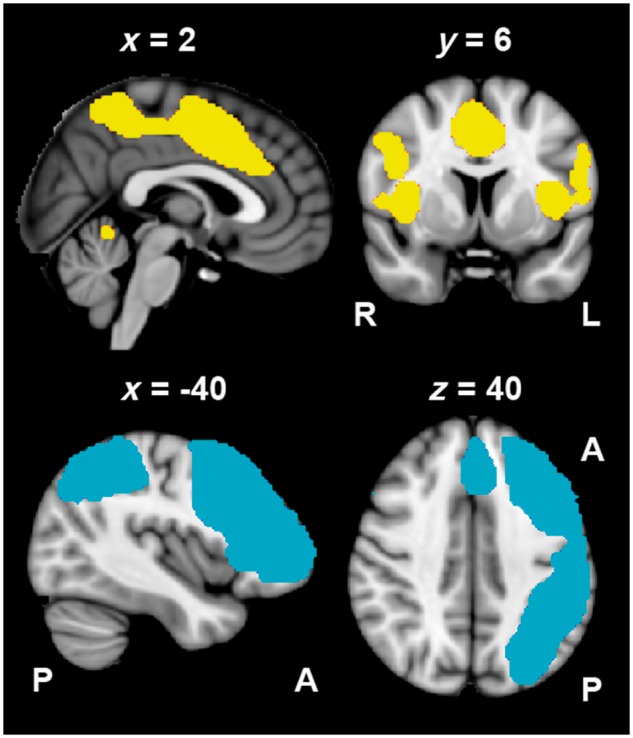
Standard T_1_-weighted anatomical slices overlaid with a mask of domain-general brain activity within cingular-opercular network (*top*) and a left-lateralized frontal-parietal-temporal language-specific network (*bottom*). The masks were obtained from previous analyses of control participants performing the same functional MRI task (Geranmayeh *et al.*, 2014b), and were used to calculate the lesion load in each network. R = right; L = left; A = anterior; P = posterior.
